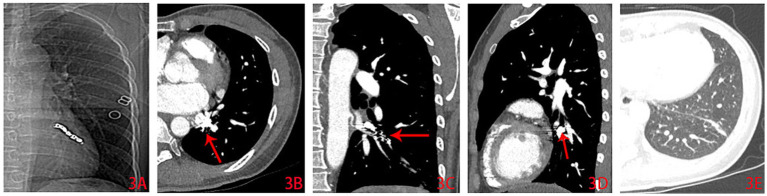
Contrast-enhanced chest CT images 2 months after embolization treatment. (A) The chest X-ray film shows the metallic shadow of the embolized coils; (B) the CT mediastinum window shows a high-density image of the metal coils; (C) the CT coronal view shows the abnormal blood vessel that have been embolized with coils; (D) the CT sagittal view shows the metal coils images in the abnormal artery; (E) 2 months after embolization treatment, the lung window shows that the shadow in the lower lateral segment of the left lung had significantly absorbed, with a few fibrous lesions left.
